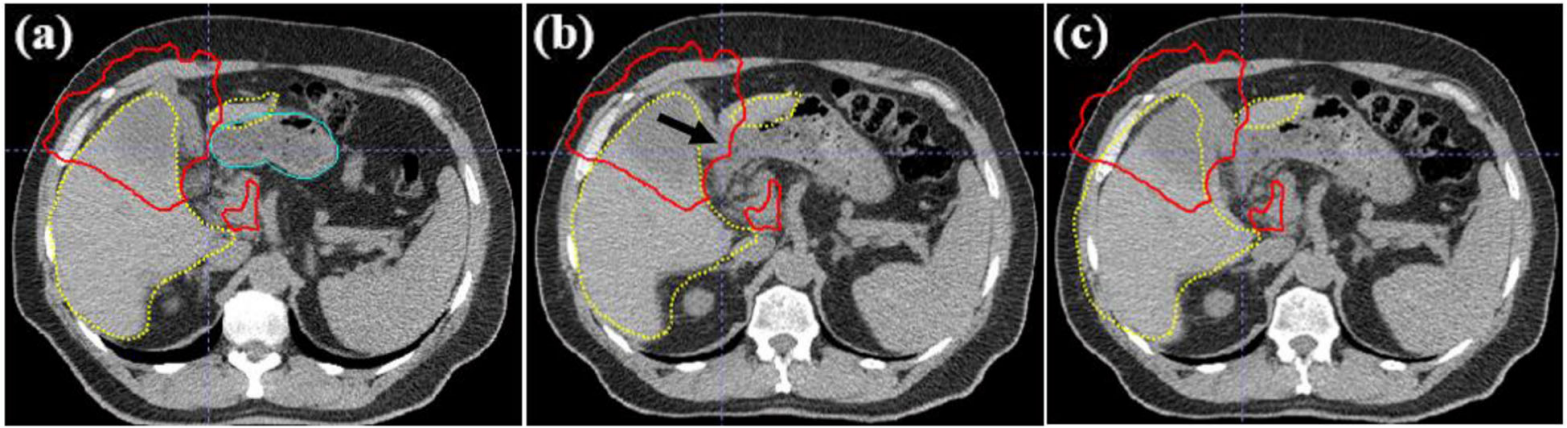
Computed tomography (CT) images showing the left-right manual shift correction of 12.7 mm. a Planning CT showing the contours of the liver (dotted yellow) and the 45 Gy isodose line (solid red) abutted the stomach (solid blue). b Result of automatic alignment showing a deformation in the shape of the stomach (black arrow). c Result of manual shift correction in which the critical dose isodose line was pulled out of the stomach
